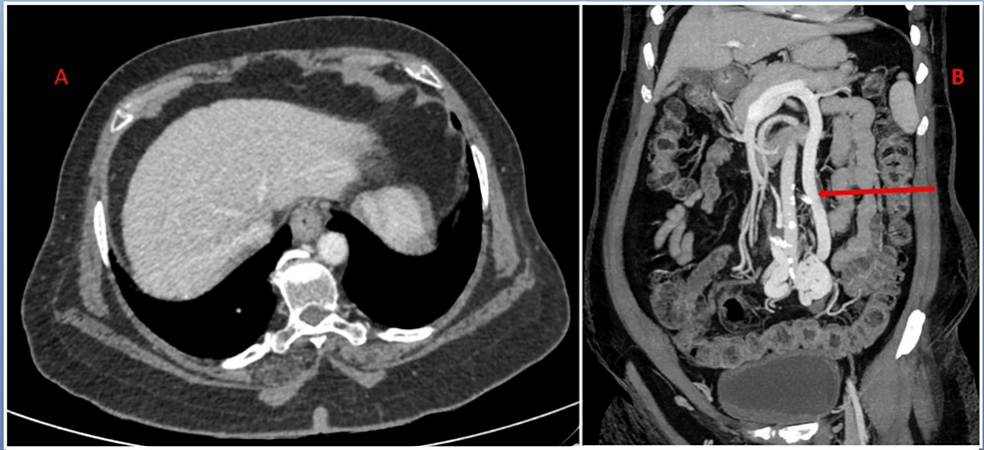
(A) Axial CT showing mild surface nodularity of the liver. (B) Coronal MIP CT image in the venous phase shows abnormal venous shunt (red arrow) connecting the extrahepatic portal vein to the left common iliac vein. CT: computed tomography; MIP: maximum intensity projection
